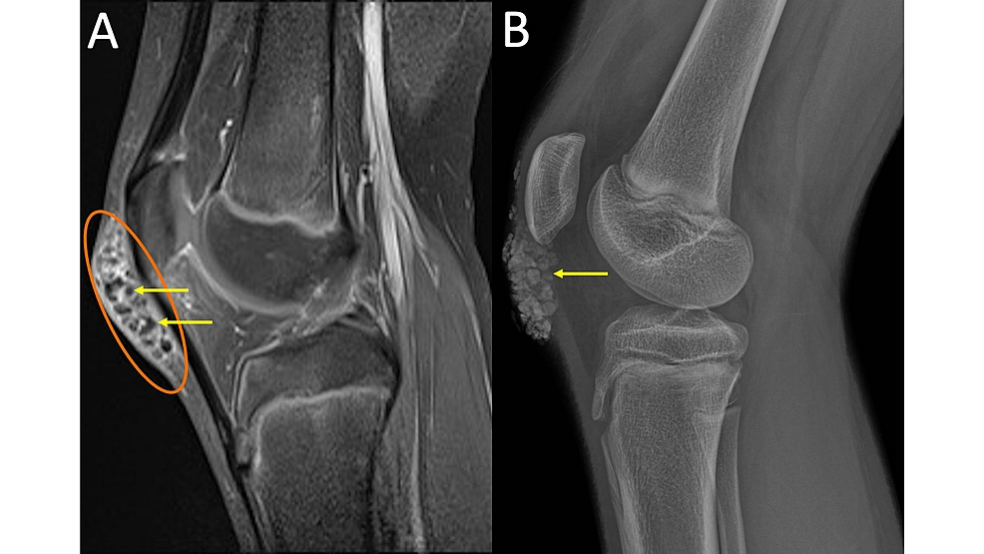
Tumoral Calcinosis MRI sagittal T1 post-contrast fat saturation (FS) of the right knee (4A) demonstrates multiple T1 hypointense rounded structures (yellow arrows) with intervening regions of enhancing synovium (orange oval) anterior to the patella and patellar tendon within the region of the prepatellar bursa. The right knee radiograph (4B) allows for easy recognition that the previously described T1 hypointense structures correspond to coarse lobulated calcifications (yellow arrow). There is no scalloping of adjacent bone nor other aggressive osseous features.
